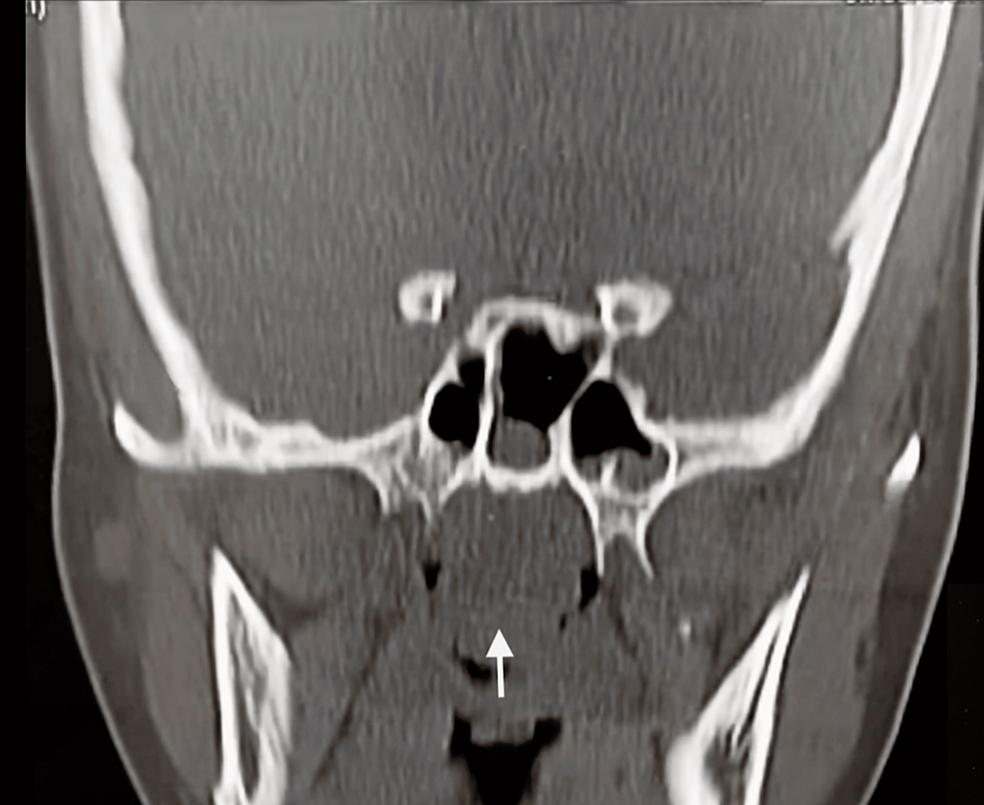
CT of the paranasal sinuses showing mucosal thickening in the right maxillary sinus and osteomeatal obstruction and soft tissue mass in the floor of bilateral nasal cavities. Arrow showing soft tissue opacity fills the choana bilaterally.
